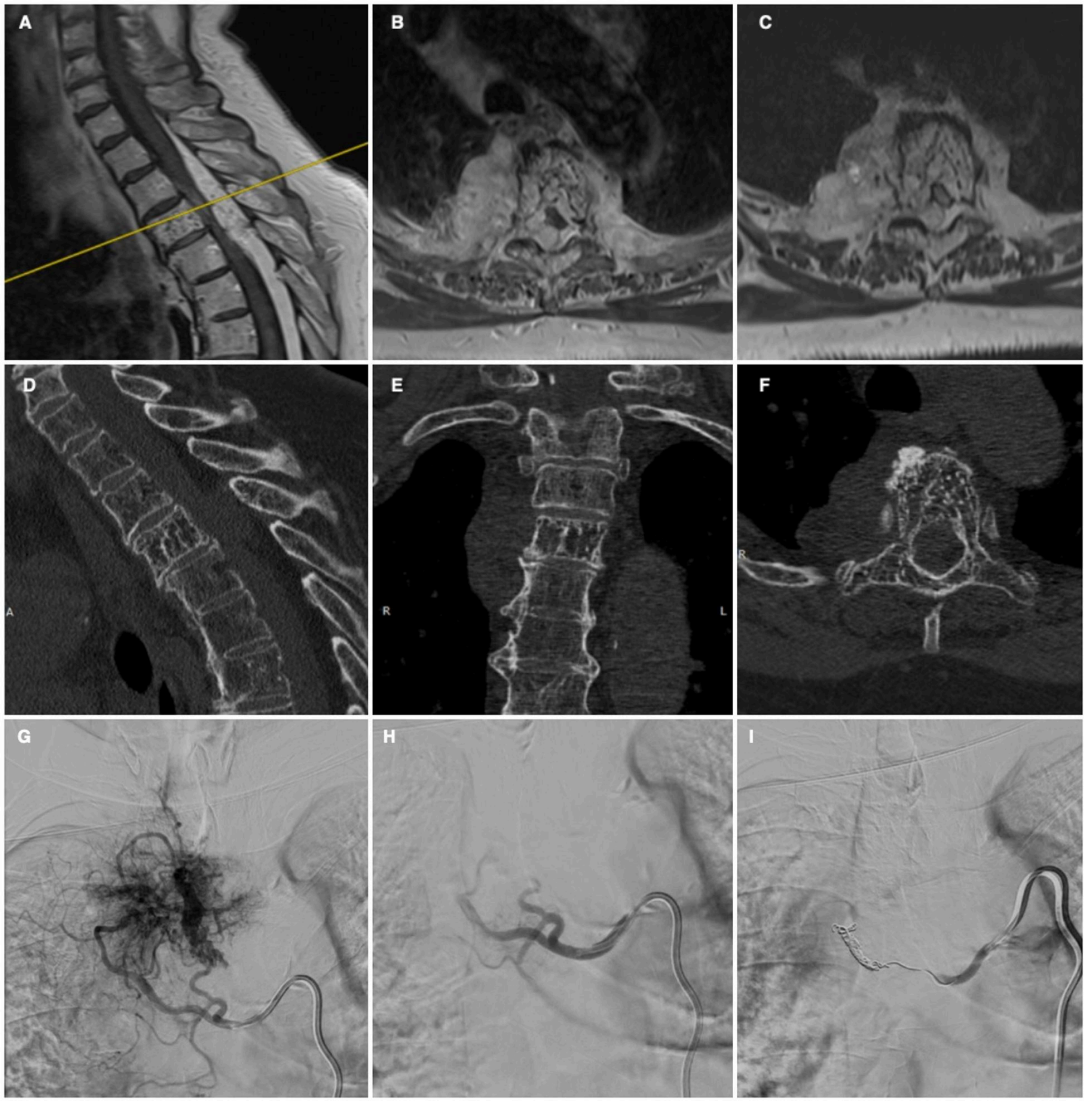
Sagittal (A) and axial (B,C) cervical-thoracic MRI images showing morpho-structural alteration of the D3 vertebral body with posterolateral involvement, associated with epidural and paravertebral pathological tissue causing compressive myelopathy. Cervical-thoracic CT images (D–F) confirming a hypervascular lesion of the D3 vertebral body with osteostructural disruption, consistent with hemangioma. Spinal angiography confirming hypervascular D3 lesion with arterial supply originating from the right D3-D4 metameric artery (G), and subsequent selective embolization using polyvinyl alcohol microparticles and microcoils (H,I).
